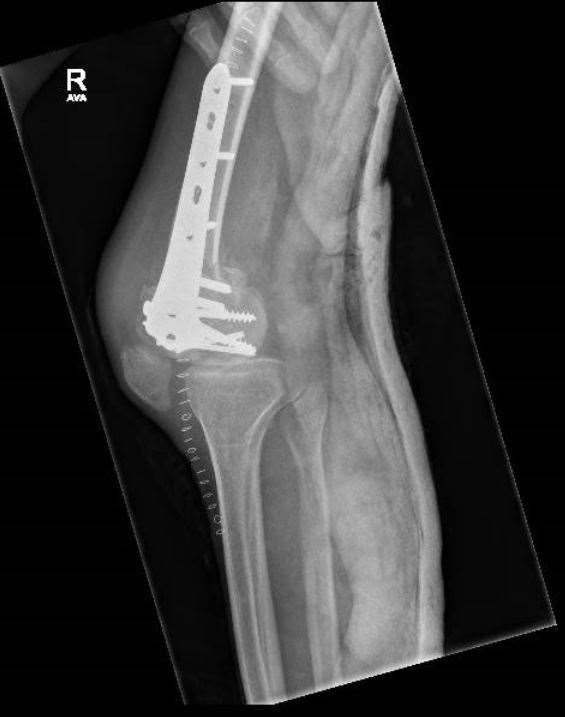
Use of multidirectional locking plate and multidirectional screws to achieve stable fixation; Lateral Radiograph.
